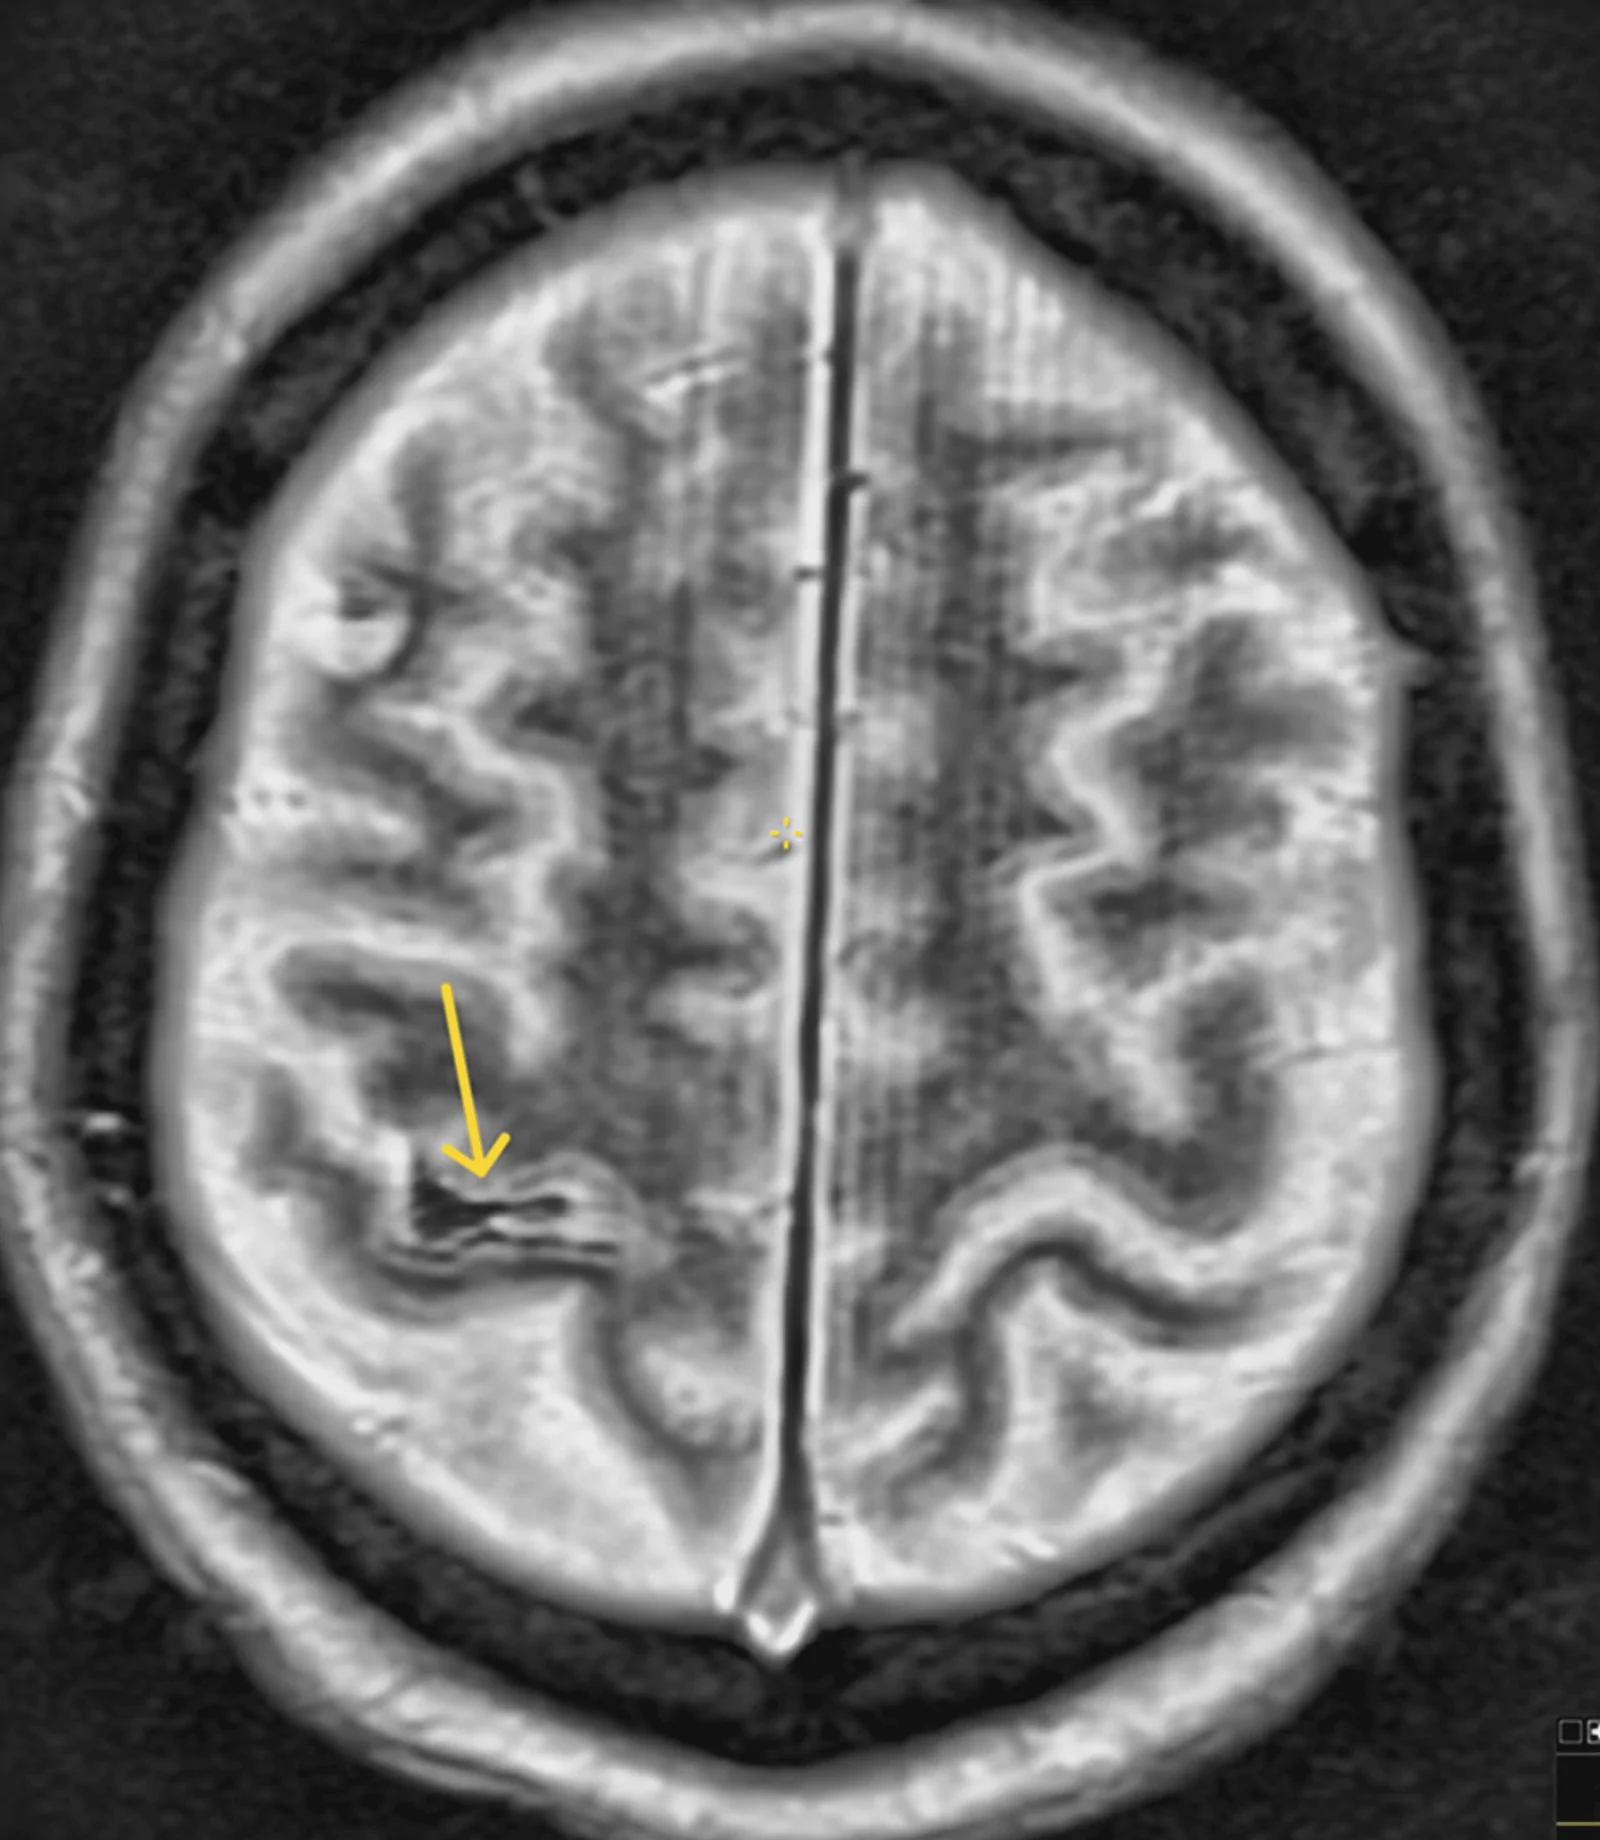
MRI of the brain on Day 4, with GRE sequence demonstrating a curvilinear band of hemorrhagic products in the high right parietal lobe (yellow arrow), compatible with hemorrhagic conversion of a subacute infarction MRI: magnetic resonance imaging; GRE: gradient-echo
